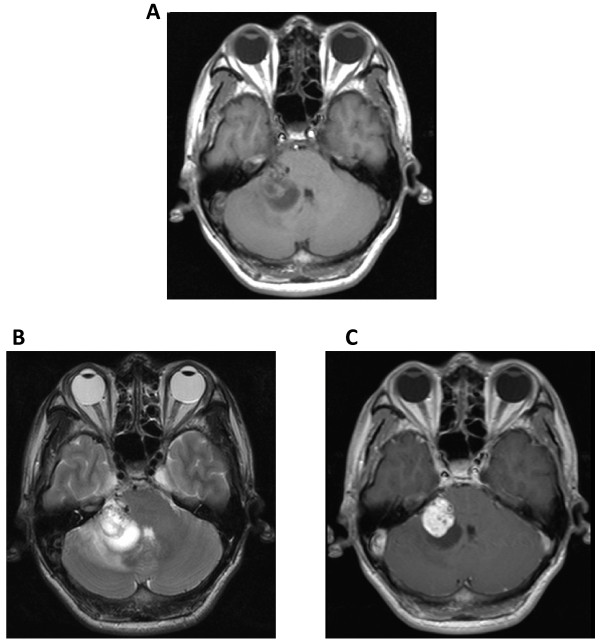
MRI showed a solid cystic lesion in the right CPA that compressed both the brain stem and the cerebellum. The anterior portion of the lesion was solid and showed signs of cystic changes, and the posterior portion of the lesion was cystic. The size of the solid component was about 2.2 cm × 2.2 cm × 2.3 cm. The solid component showed hyper- and isointensity on T1WI images (A) and mixed intensity on T2WI images (B), and it was significantly enhanced after contrast administration (C).
